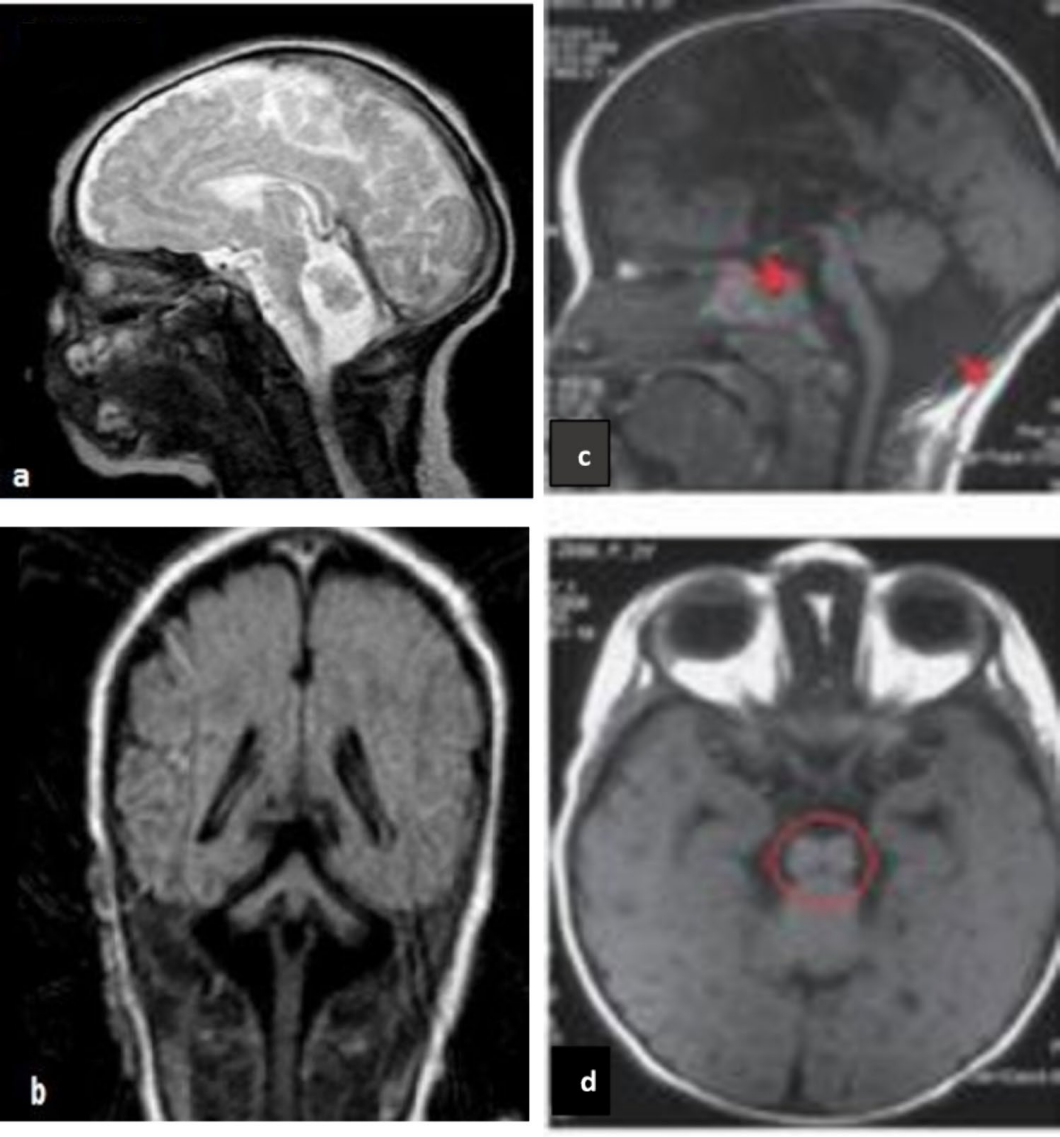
(a-d) Typical radiological findings shown—(a, b) dragonfly pattern (comparatively preserved vermis and atrophic cerebellar hemispheres) in one patient with TSEN54 gene. (c, d) brainstem appearance of figure of 8 on the brain MRI of one patient with AMPD2 gene
